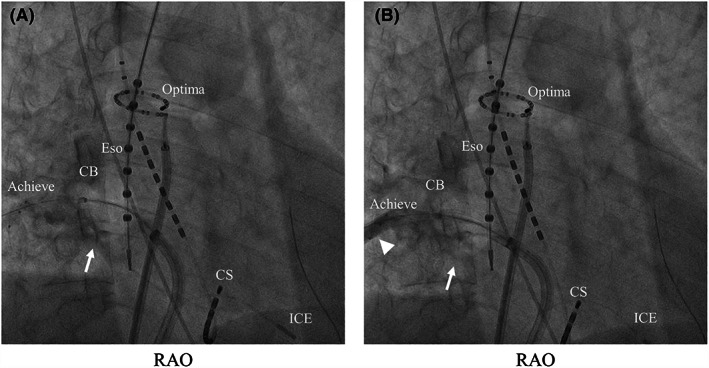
Right inferior pulmonary vein (RIPV) cryoballoon (CB) ablation with conventional method and balloon lever technique (BLT) in a representative patient. The achieve catheter is inserted into the inferior branch. (A) Massive leakage of the contrast medium is observed at the inferior site of the RIPV (white arrow). The shape of the RIPV could not be confirmed due to the flushing of the contrast medium. (B) Optimal RIPV occlusion is achieved with the BLT. The CB is shifted downward and pressed to the bottom of the RIPV (white arrow). A selective contrast injection to the RIPV shows contrast retention, and the shape of the RIPV could be discerned (white arrowhead). The optima is positioned in the superior vena cava. An intracardiac echocardiography catheter (ICE) is positioned in the right ventricle. CS, coronary sinus; Eso, esophageal temperature monitoring catheter; RAO, right anterior oblique projection.
